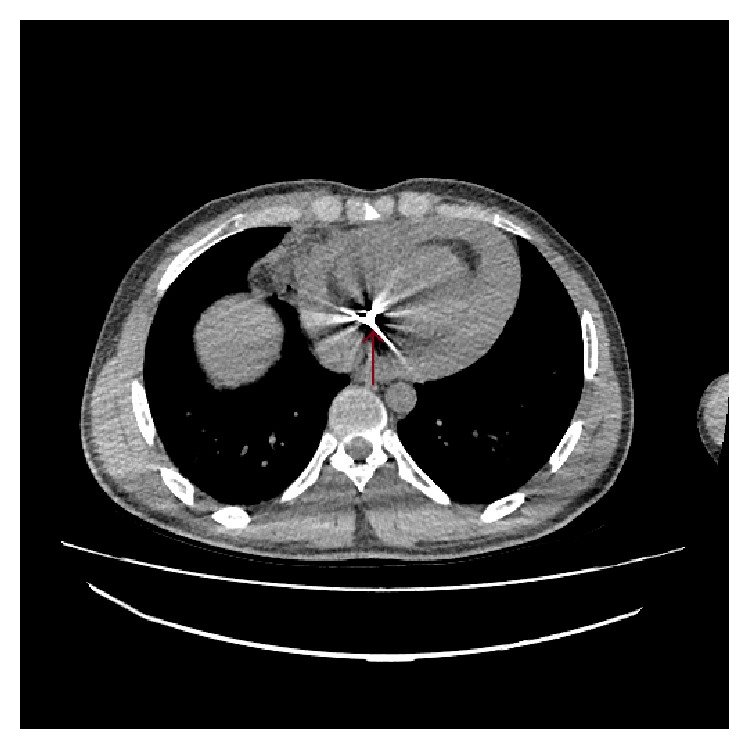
Transverse cut view of chest CT shows a bullet in the pericardial sac with pericardial effusion.
